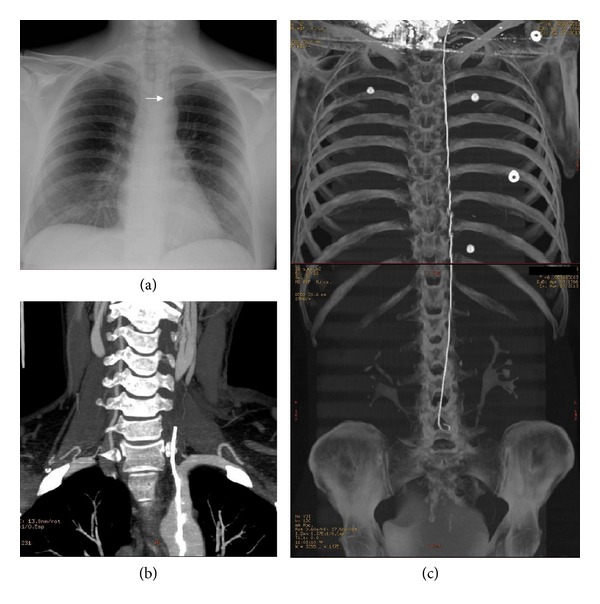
(a) Chest X-ray revealing the presence of the guide wire in the left hemithorax (arrow). (b) Cervical CT angiography showing insertion of the guide wire in the left subclavian artery, causing left vertebral artery occlusion, with the tip inserted in the superficial neck tissues. (c) Total body CT showing the vascular course of the guide wire as far as the distal abdominal aorta.
